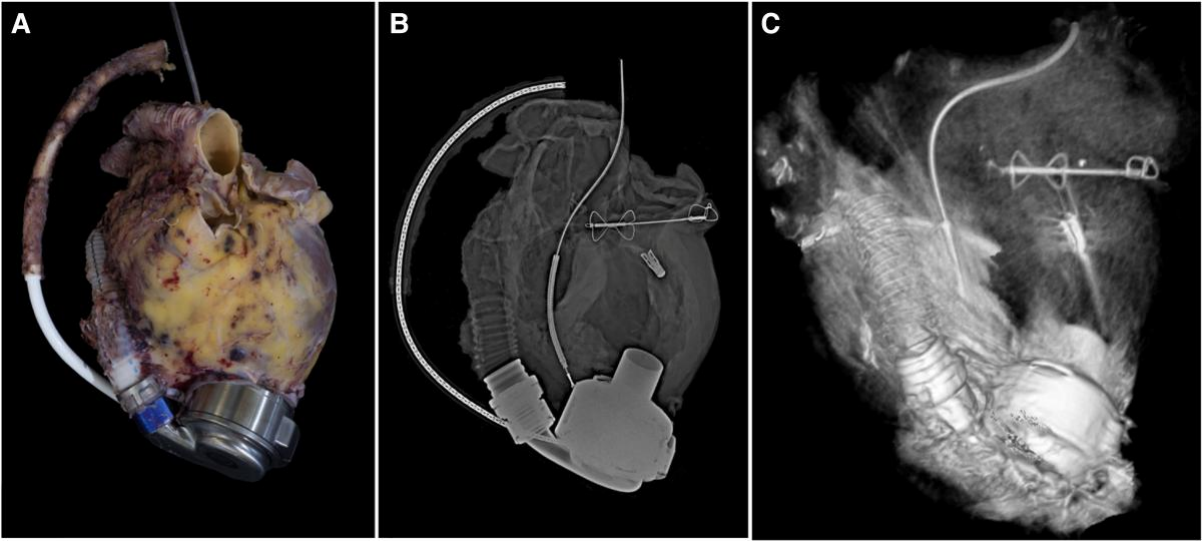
Gross anatomy of removed heart and associated supportive devices following heart transplantation. Image *A* is the gross pathologic images with corresponding radiographic (*B*) and CT reconstruction (*C*).
